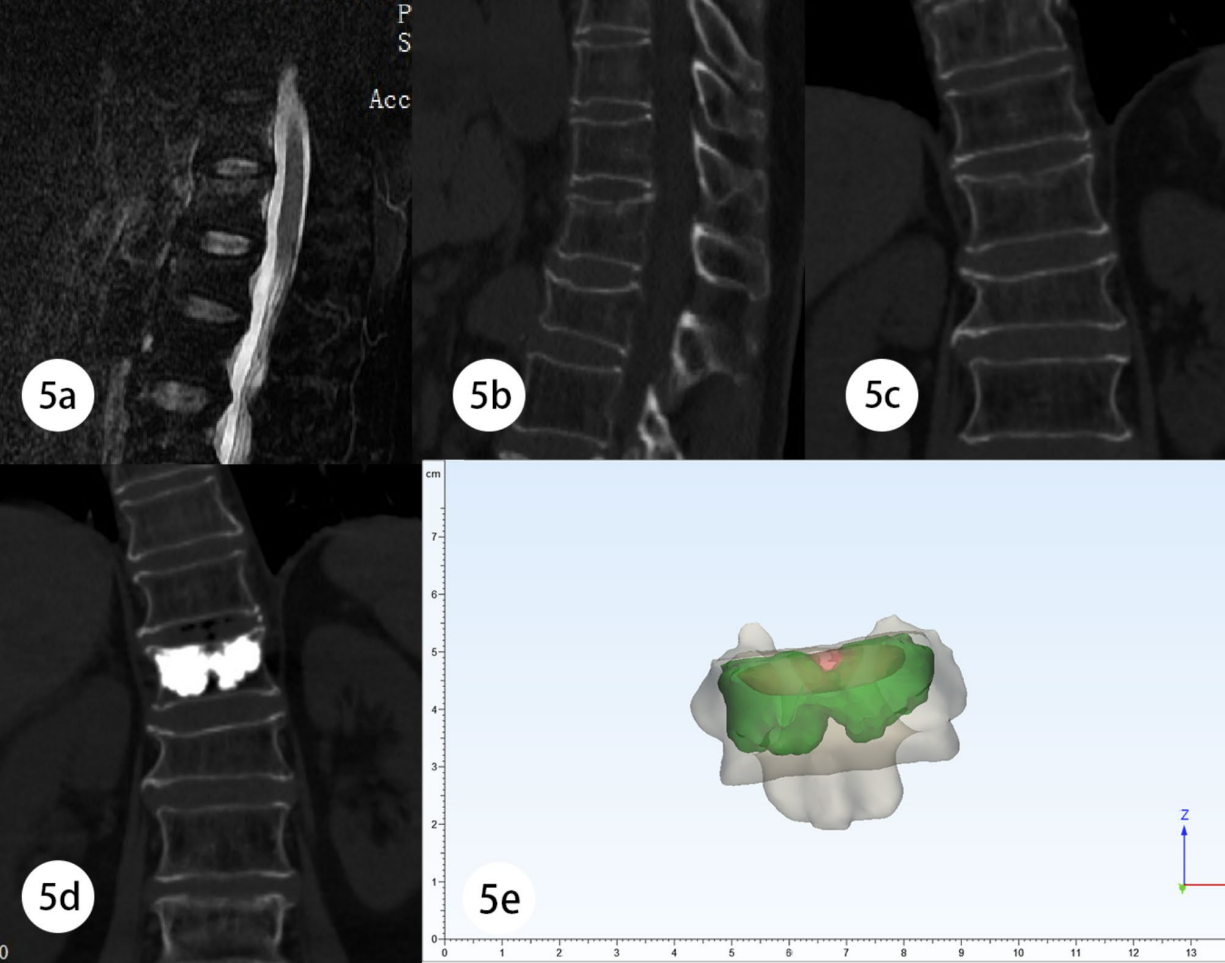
Case2: 5a The preoperative fat suppression image of thoracolumbar spine MRI showed SNs with Modic I changes in the cephalic endplate of T12 vertebra. 5b、c The sagittal and coronal images of preoperative CT. 5d Postoperative CT showed excellent distribution of bone cement. 5e Reverse reconstruction model, calculated R_f_=90.12%
